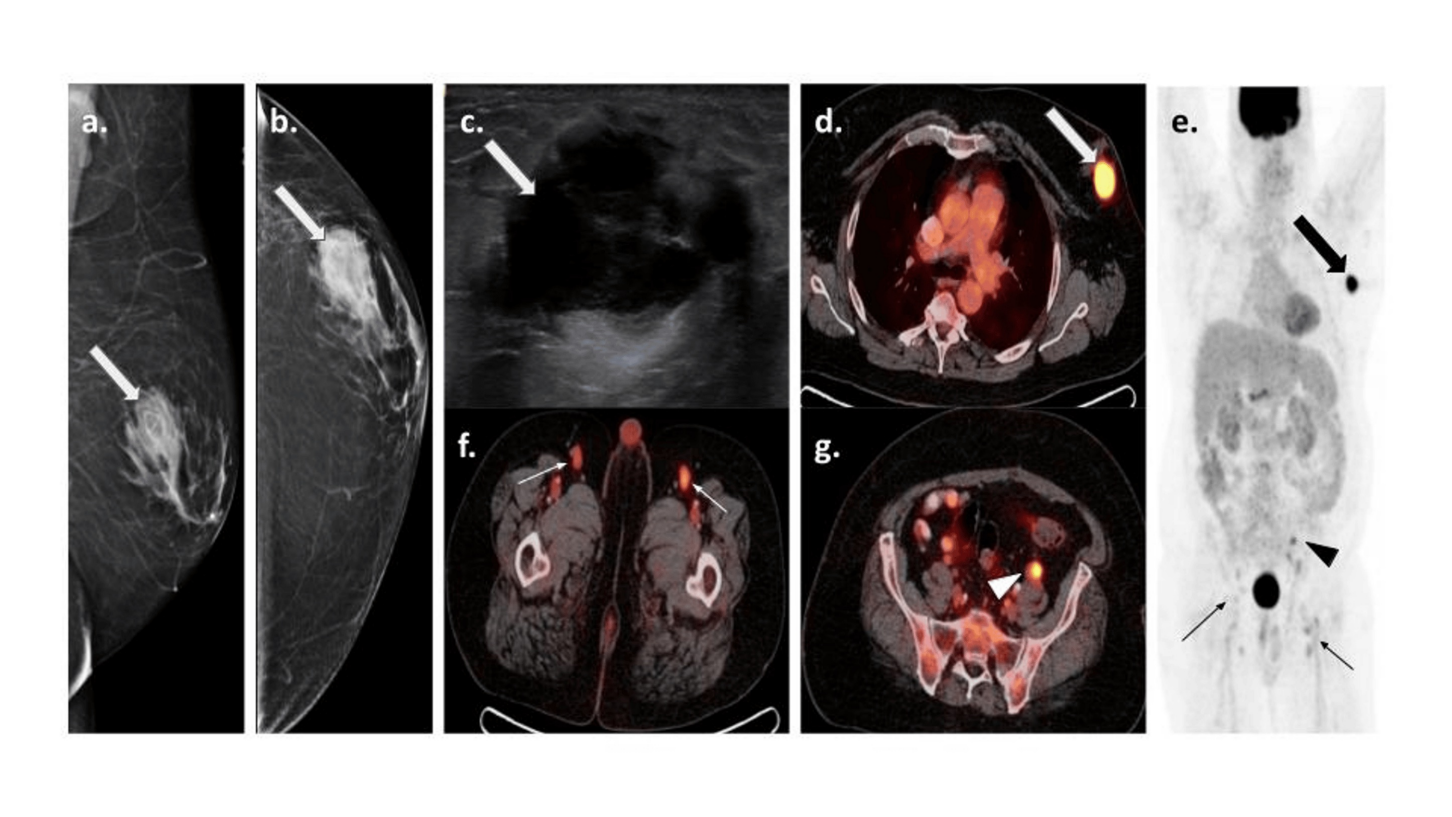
A 61-year-old male presented with a palpable left breast mass. Mediolateral oblique (MLO) (a) and cranio-caudal (CC) (b) views of mammogram show a mass (white arrows) in the outer central left breast at 3:00 o’clock, 2 cm from the nipple. Targeted grayscale ultrasound image of the left breast (c) shows a complex solid and cystic mass (white arrow) with thick internal septations. Ultrasound-guided biopsy yielded DLBCL. (d-h) PET/CT images show the metabolically active (SUV max 17.86) left breast mass (white arrow on (d) and black arrow on (e)), correlating to the biopsied lymphoma. Additionally, there are multiple metabolically active left common iliac (SUV max 4.57) (black arrowhead on (e) and white arrowhead on (g)) and bilateral inguinal (SUV max 4.73) (thin black arrows on (e) and thin white arrows on (f)) lymph nodes.
